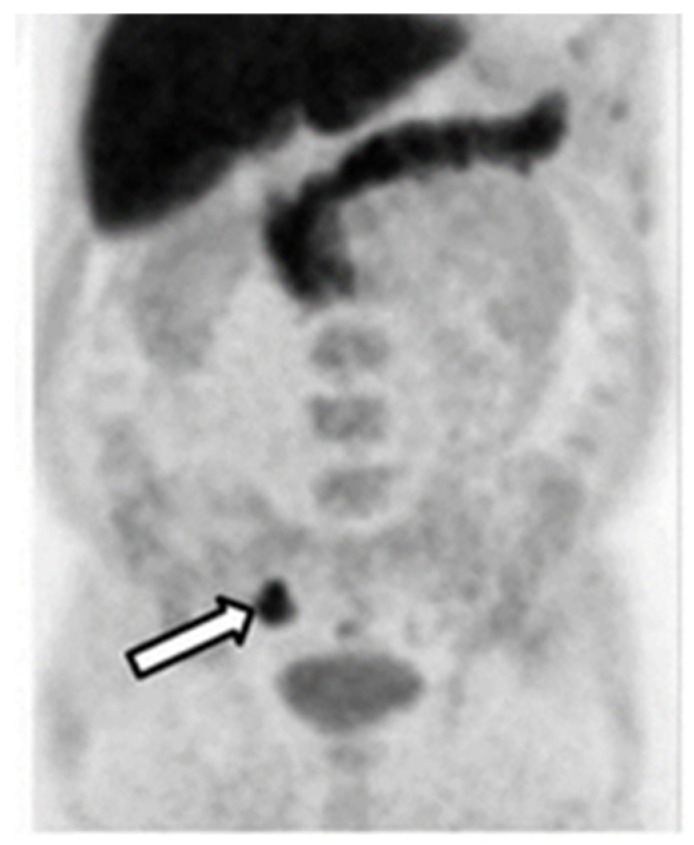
A [^18^F]fluciclovine PET/CT image of PCa (white arrow). This research was originally published in the Journal of Nuclear Medicine (JNM) [36].
